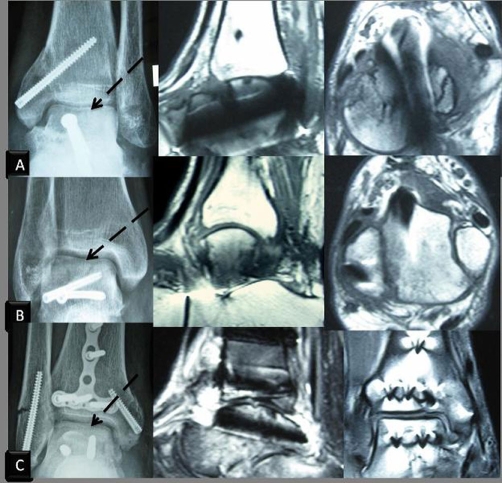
Anteroposterior radiographs (8 weeks post-op) and MRI scans (last follow-up) of patients. Anteroposterior radiographs of patients following talar body fracture, with Hawkins' sign (subchondral radiolucent band) was observed in the talar dome 8 weeks postoperatively (arrow) and MR scans of last follow-up. (A) Case 1; (B) Case 2; (C) Case 3.
